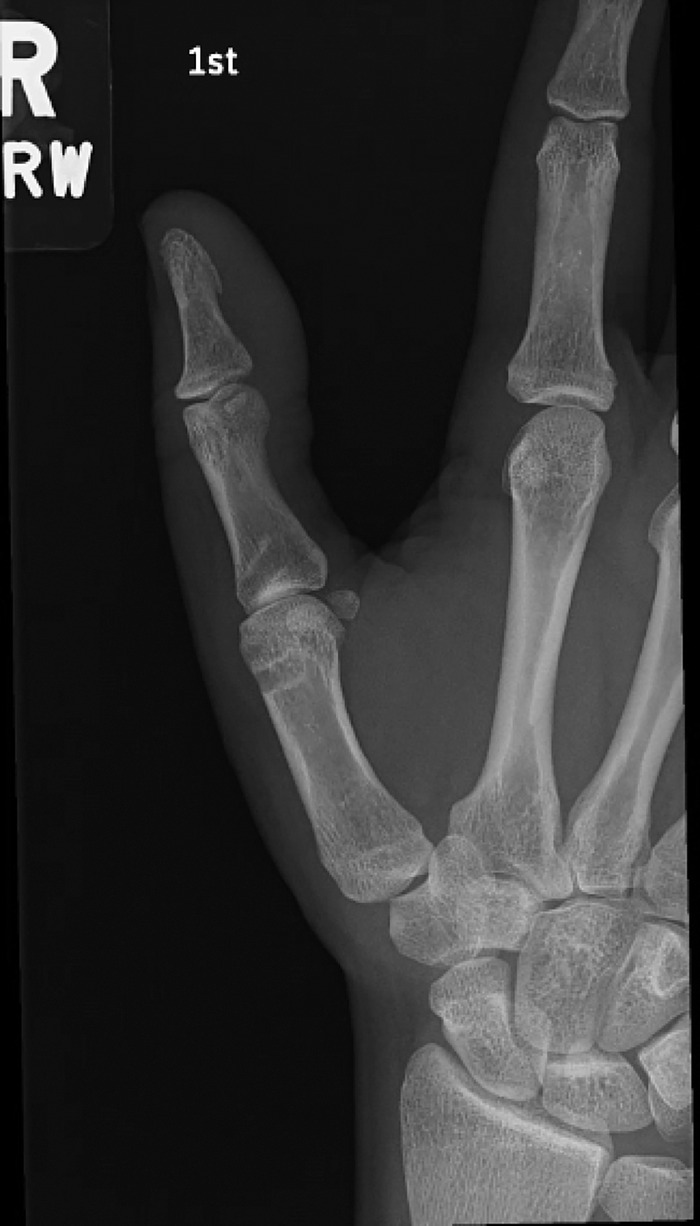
Postoperative radiograph of the right thumb shows restored alignment of the metacarpophalangeal joint.
